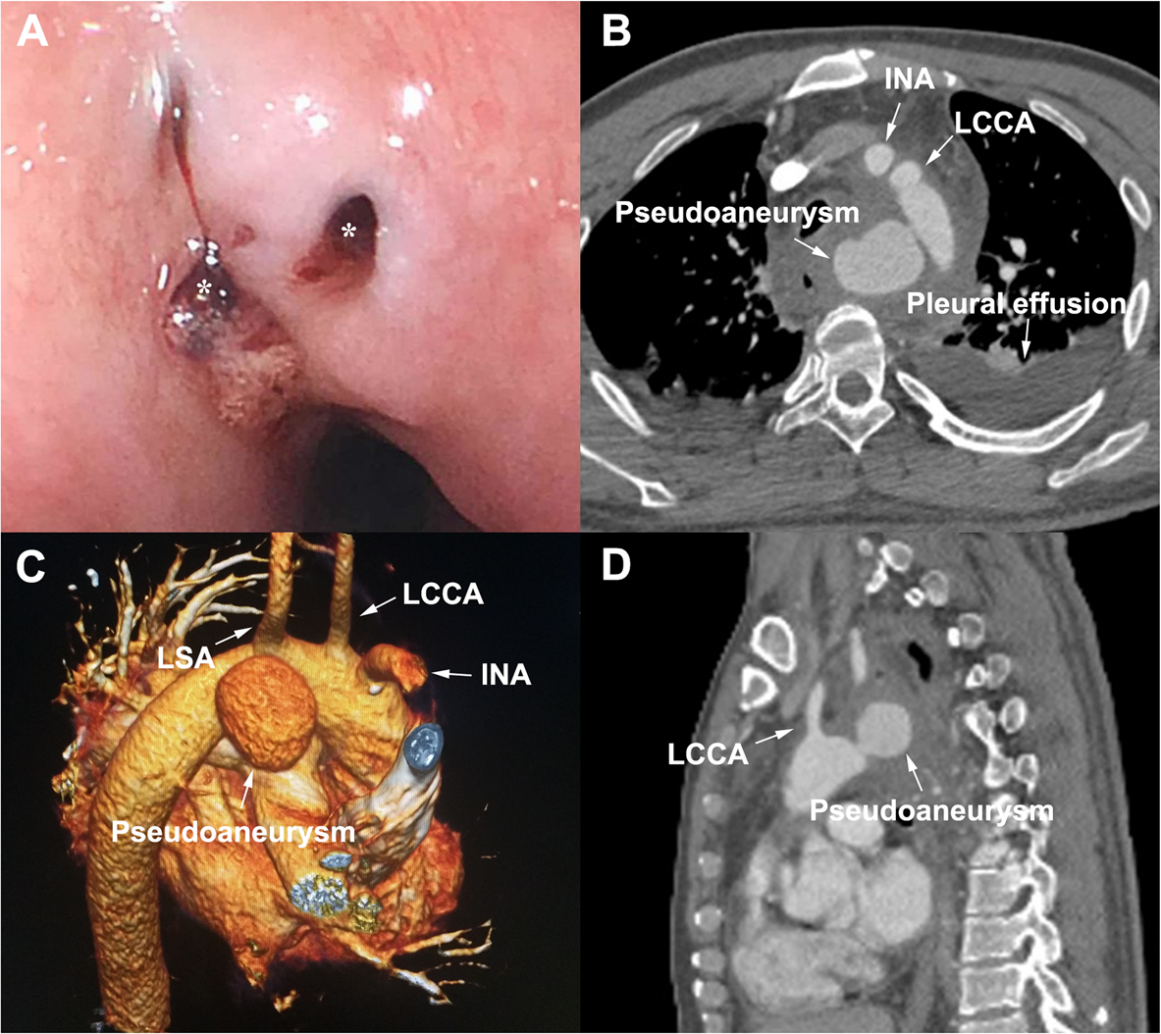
The pre-surgical examination: (a) Gastroscopic examination showed two esophageal fistulas approximately 0.2 cm and 0.3 cm in diameter respectively were found 23 cm away from the incisors; (b) Transverse, (c) three-dimensional volume-rendered and (d) sagittal computed tomography angiography demonstrated the pseudoaneurysm with a primary entry tear at the arch accompanied by gas-containing pleural effusion. *, irregular esophageal mural ulcers; LSA, left subclavian artery; LCCA, left common carotid artery; INA, innominate artery
